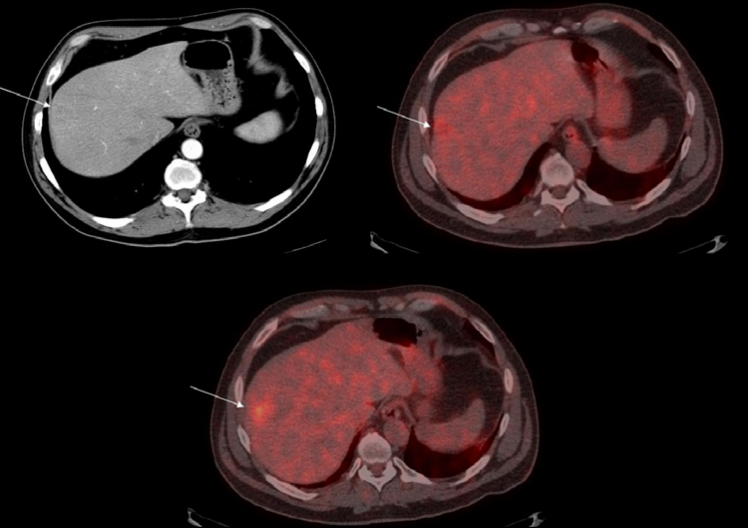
CECT (top left), early(top right) and delayed phase (bottom) FDG-PET/CT fusion images of a 46 year old male with lung carcinoma showing delayed accumulation of FDG in a segment 7 metastatic lesion.
